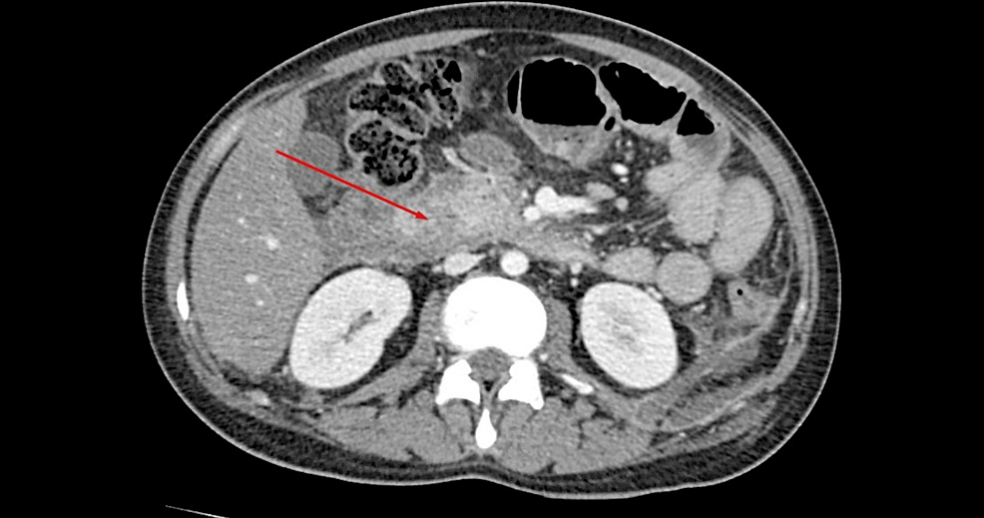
Contrast-enhanced CT of the abdomen showing features consistent with acute pancreatitis, including peripancreatic fluid collection (indicated by the red arrow)
